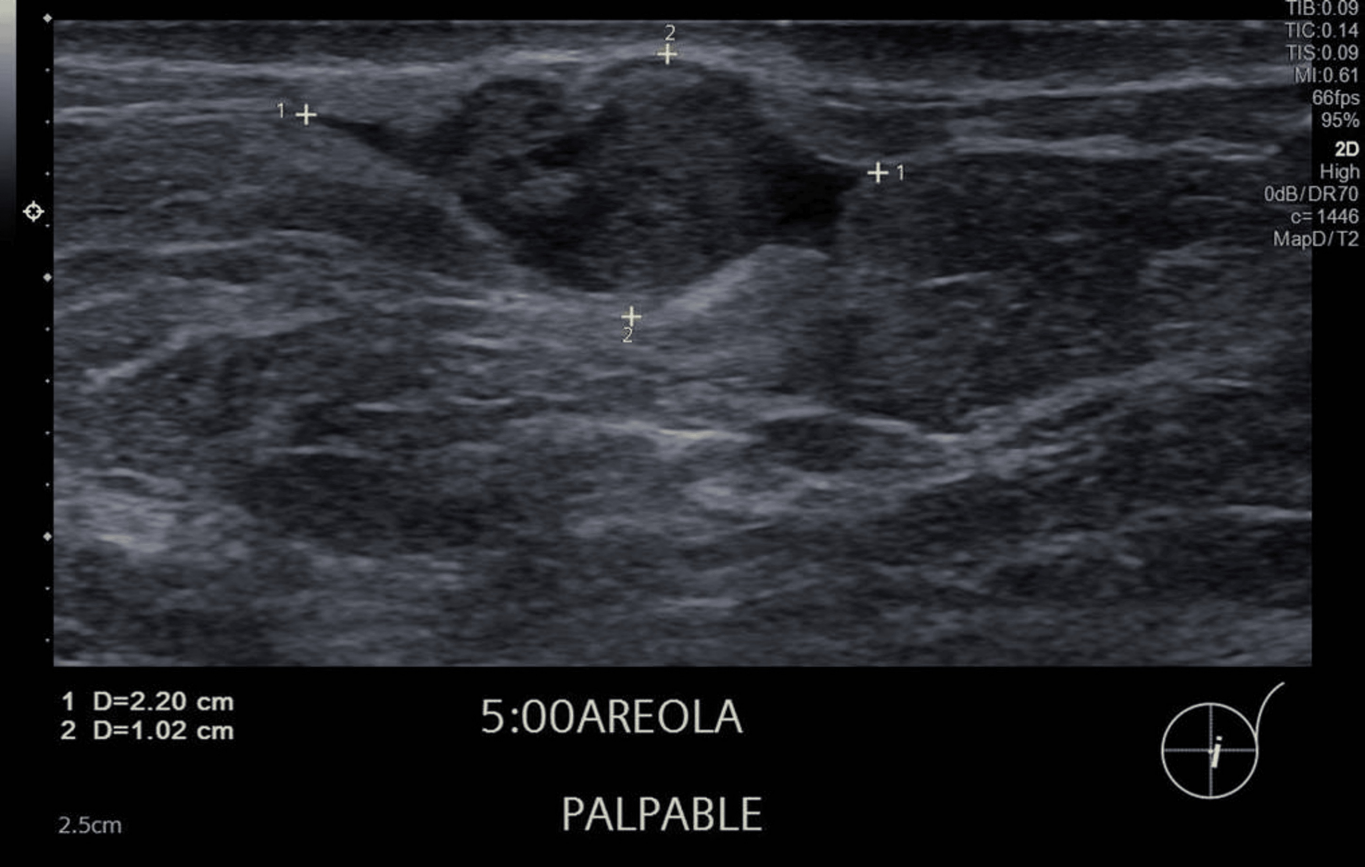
Left breast lesion under the skin Ultrasound showed a lobulated hypoechoic heterogenous nodule with small cystic areas, at 5 o'clock subareolar region under the skin in the left breast measuring 2.2 cm.
